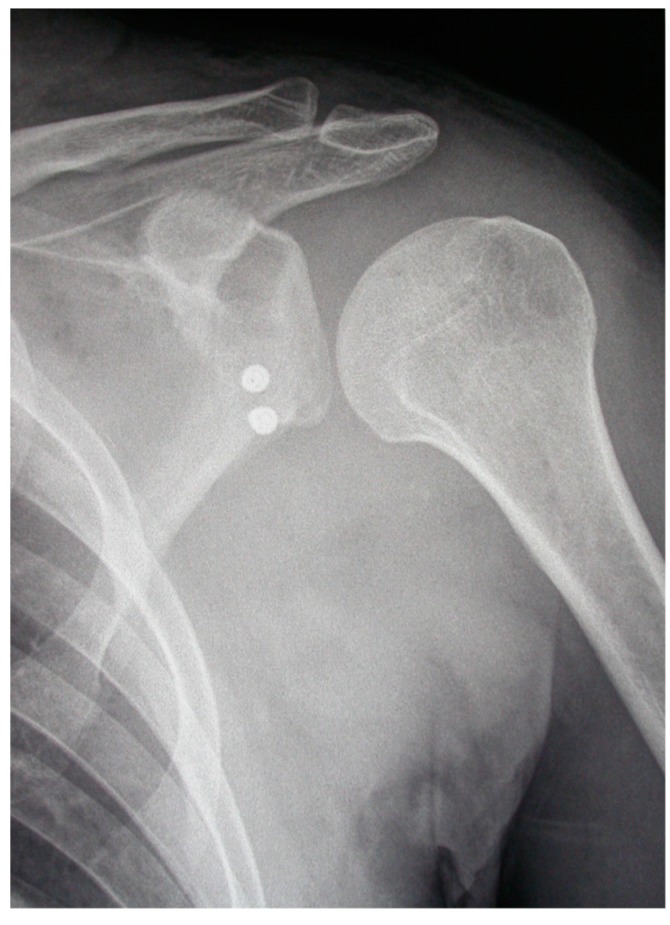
Postoperative X-ray control showing that the graft has perfect positioning.
